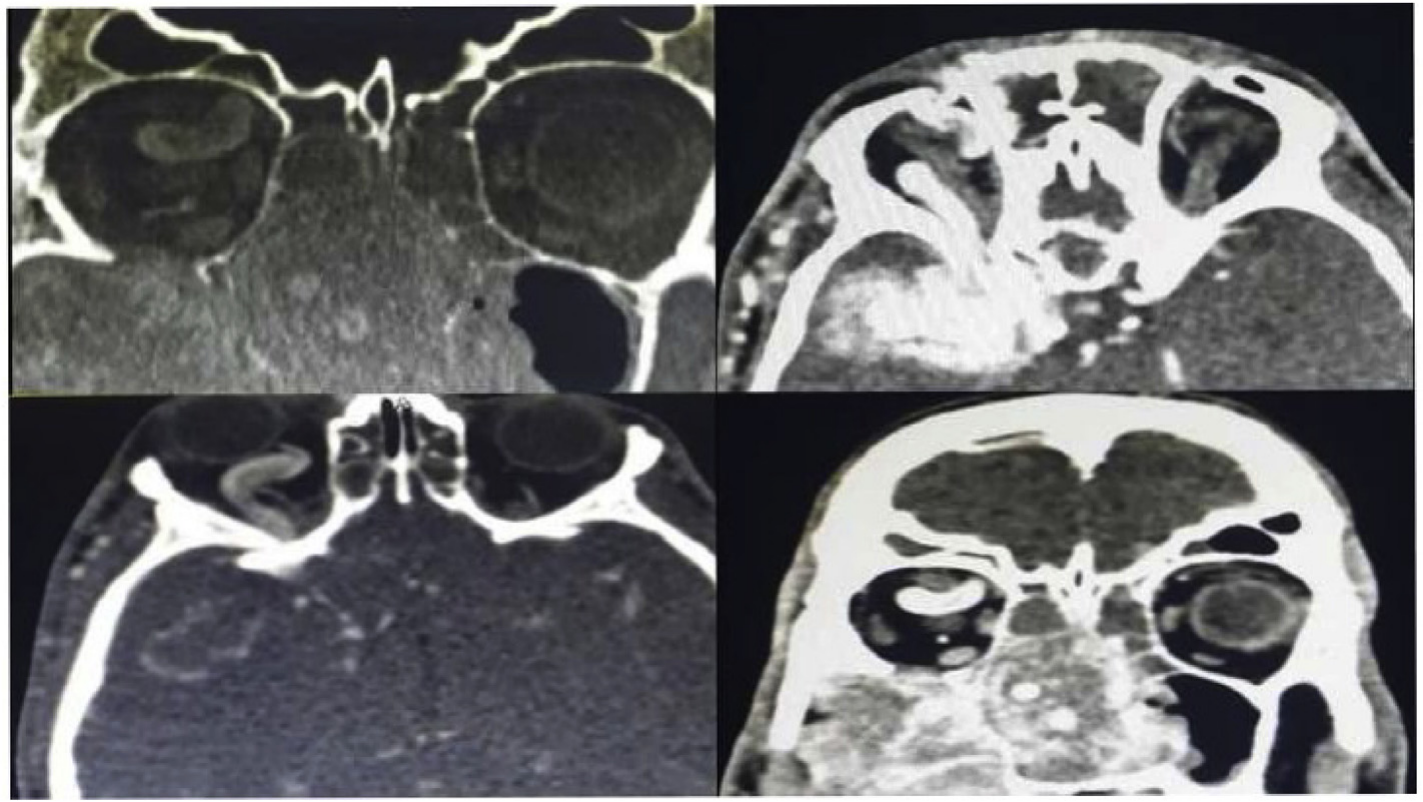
The computed tomography scan with intravenous contrast revealed a large hypervascular mass in right nasal cavity with orbital involvement.
